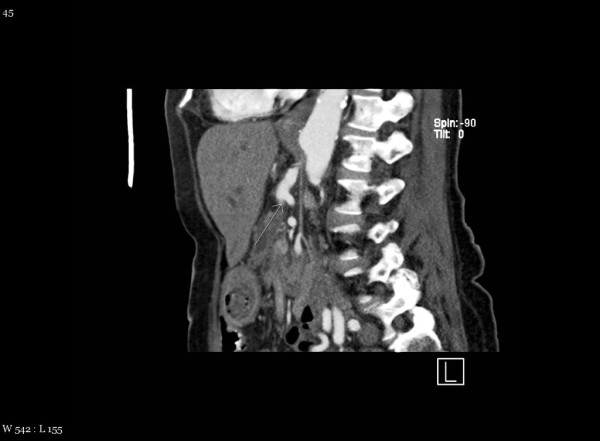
CT demonstrating abnormal celiac origin.
